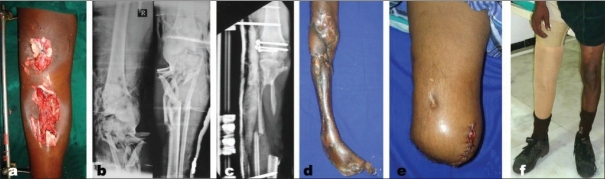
(a) Clinical photograph shows severe open injury of tibia with soft tissue loss. (b) X-rays (anteroposterior view) showing severe communition of the bones in proximal 1/3rd of tibia. (c) X-ray (anteroposterior view) showing gap non-union upper end tibia. (d) Clinical photograph of same patient showing an infected non-union with sinuses and a deformed foot. (e) Clinical photograph of same patient showing above-knee amputation. f) Clinical photograph after rehabilitation
